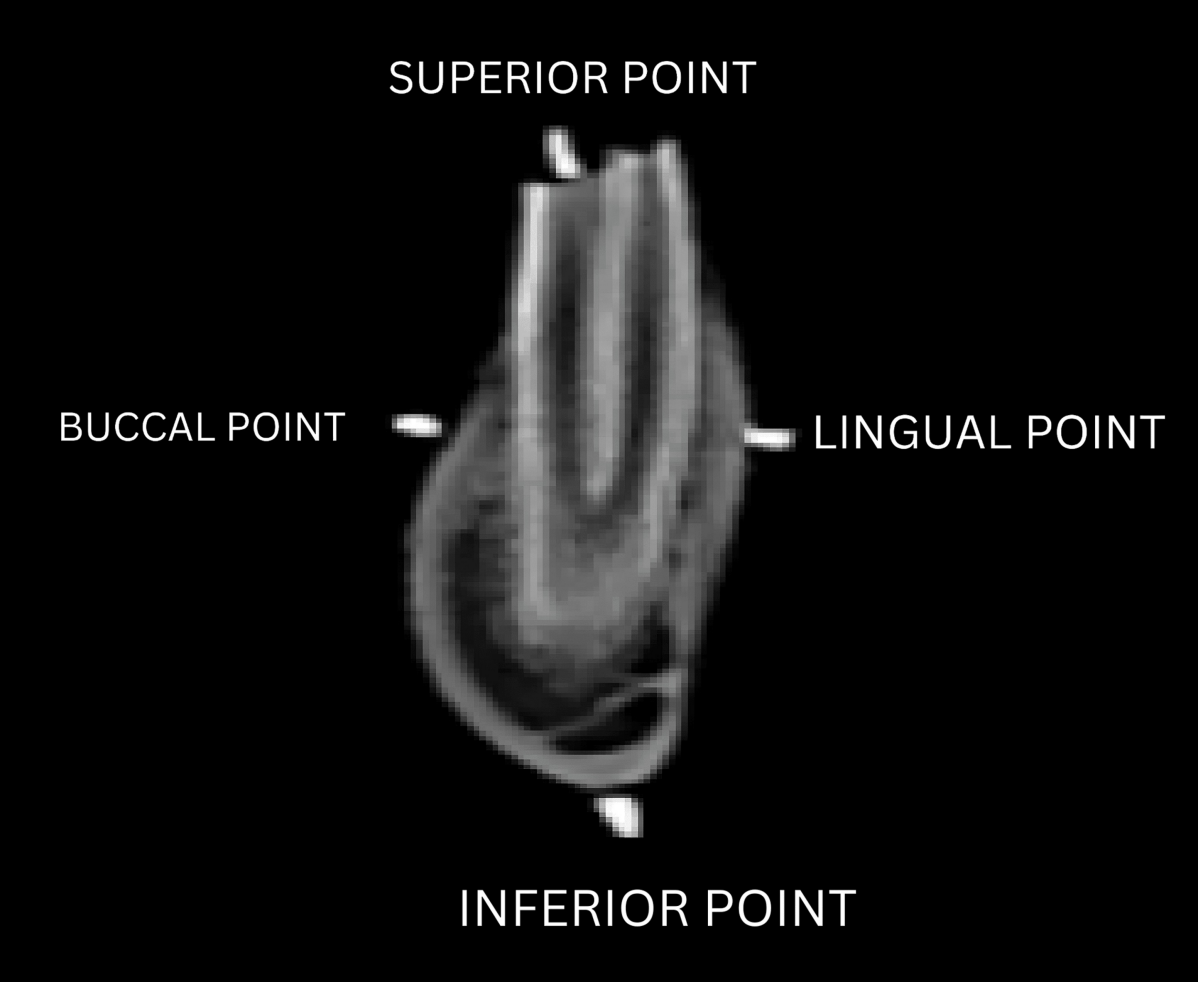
Cross-section of the site of interest taken from the CBCT scan showing the delineation of the mandible The superior and inferior points mark the path of measurement for the mandibular height. The buccal and lingual points mark the path of measurement for the mandibular width. CBCT: cone-beam computed tomography
